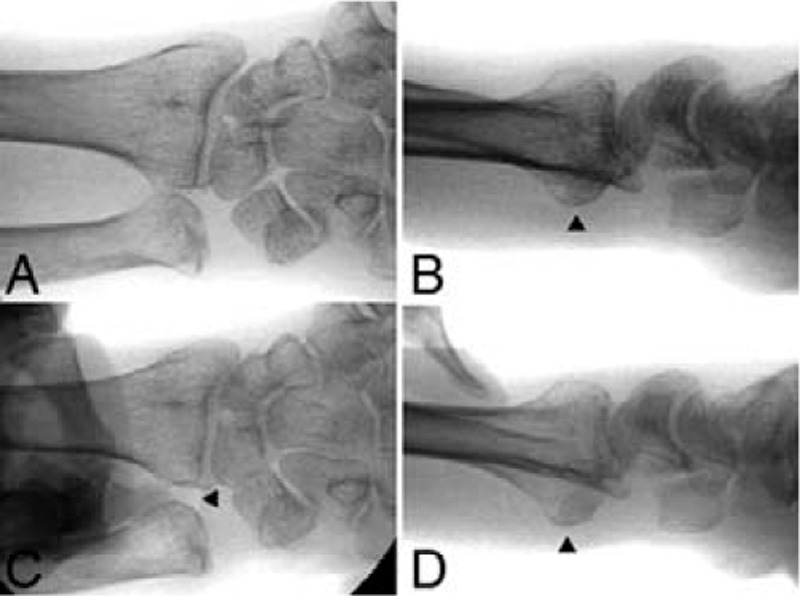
Perioperative radiographs of the left wrist. Anteroposterior (A), (C) and lateral (B), (D) views. Without stress, there is no diastasis of the DRUJ on the anteroposterior view (A), but the ulnar head is subluxated volar to the radius on the lateral view (B) (arrowhead). A manual stress traction test of the ulna shows marked diastasis of the distal radioulnar joint on the anteroposterior view (C) (arrowhead), and a volar press-down stress test of the ulnar head shows that the ulnar head is easily dislocated volarly to the radius on the lateral view (B) (arrowhead).
